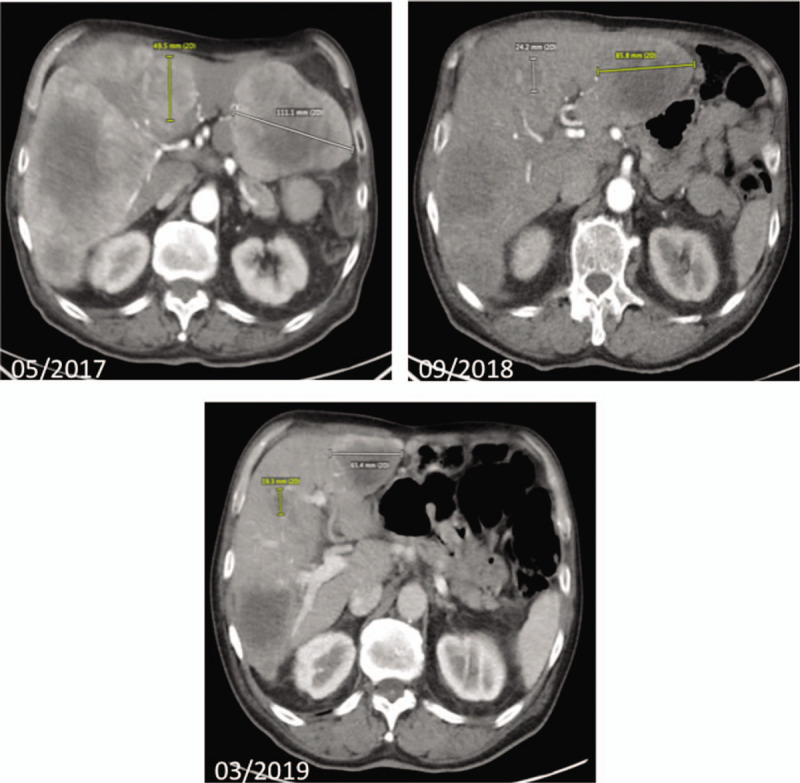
Hepatic metastases in abdominal CT - imaging before (05/2017) and during (09/2018, 03/2019) treatment with IFNα2a. CT imaging before initiation of treatment (05/2017) depicts a hepatic tumor load of 70%, whereas CT imaging in march 2019 shows a significant reduction of hepatic tumor load to 30% (03/2019). CT = computed tomography.
